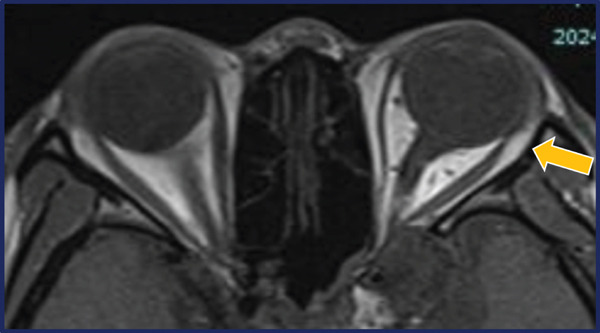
Orbital MRI at 5 months postoperatively. The myectomized left lateral rectus muscle is shown to be reattached to the posterior globe via fibrous tissue. The arrow indicates the site of posterior reattachment.
